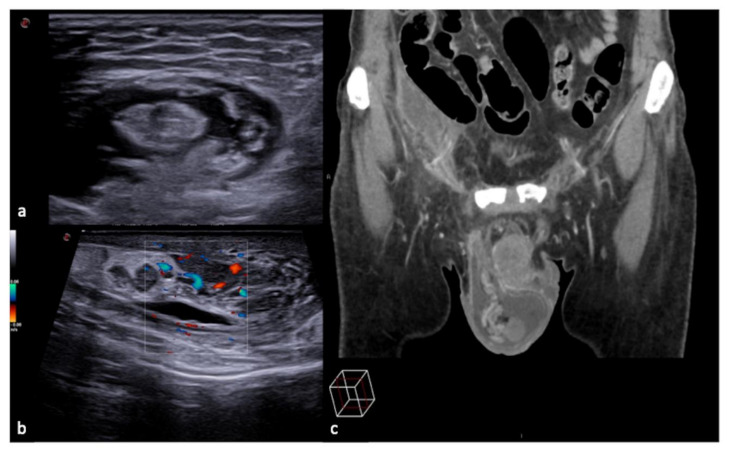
Longitudinal US B-mode (a) and color-Doppler (b) scan of the inguinal canal show a blockage of the inguinal canal misinterpreted as an inguinal hernia with congested intestinal loop. On CT scan (c) it appears to be a right epididymitis with funiculitis.
